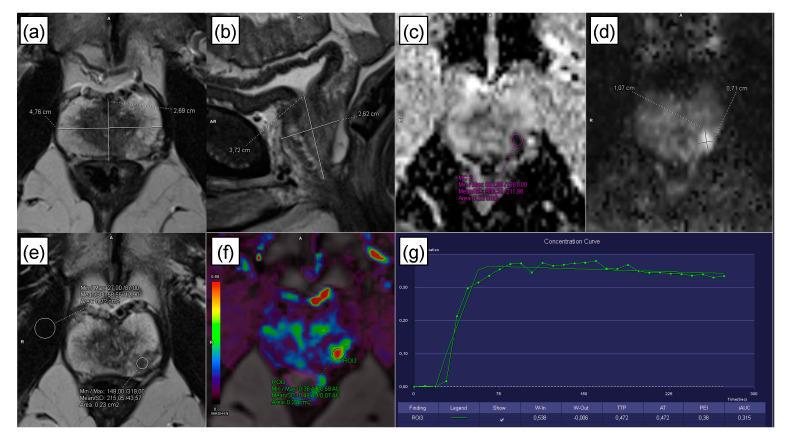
Image assessment. (a,b) Prostate volume was calculated from the diameters measured in axial and sagittal T2w images, as described in the PI-RADS v2.1 guidelines [6]. A circular region of interest (ROI) was placed within a lesion, for lesions of the peripheral zone, preferably on the ADC map (c) in additional consideration of DWI (calculated b-value of 1500 s/mm^2^; (d)), and for lesions of the transitional zone/central gland, preferably in the axial T2w sequence (e) [5,6]. Following placement, the ROI was copied to the other sequences using the software. If lesion measurement was difficult or compromised on ADC (for peripheral zone lesions) or T2w (for transitional zone/central gland lesions), measurements were performed on the sequence that showed the lesion best [5,6], e.g., in the above example, lesion dimensions were assessed on DWI (d). (f,g) Time-intensity curves were automatically calculated by the software, yielding the dynamic parameters: wash in, wash out, time to peak (TTP), peak enhancement intensity (PEI), arrival time (AT), and initial area under the curve (iAUC).
